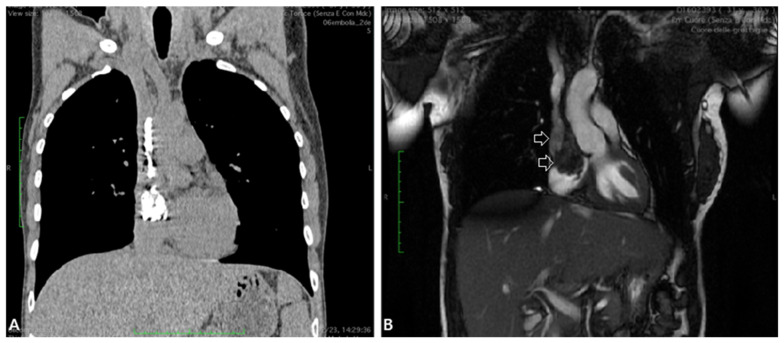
Case 1. (A) AngioCT, coronal view, showing the cardiac calcified amorphous tumor (CAT) in the superior vena cava and in the right atrium; (B) cardiac magnetic resonance image, coronal plane, showing the same lesion (arrows), with clear delineation of its intracardiac extent and relationship to adjacent structures.
